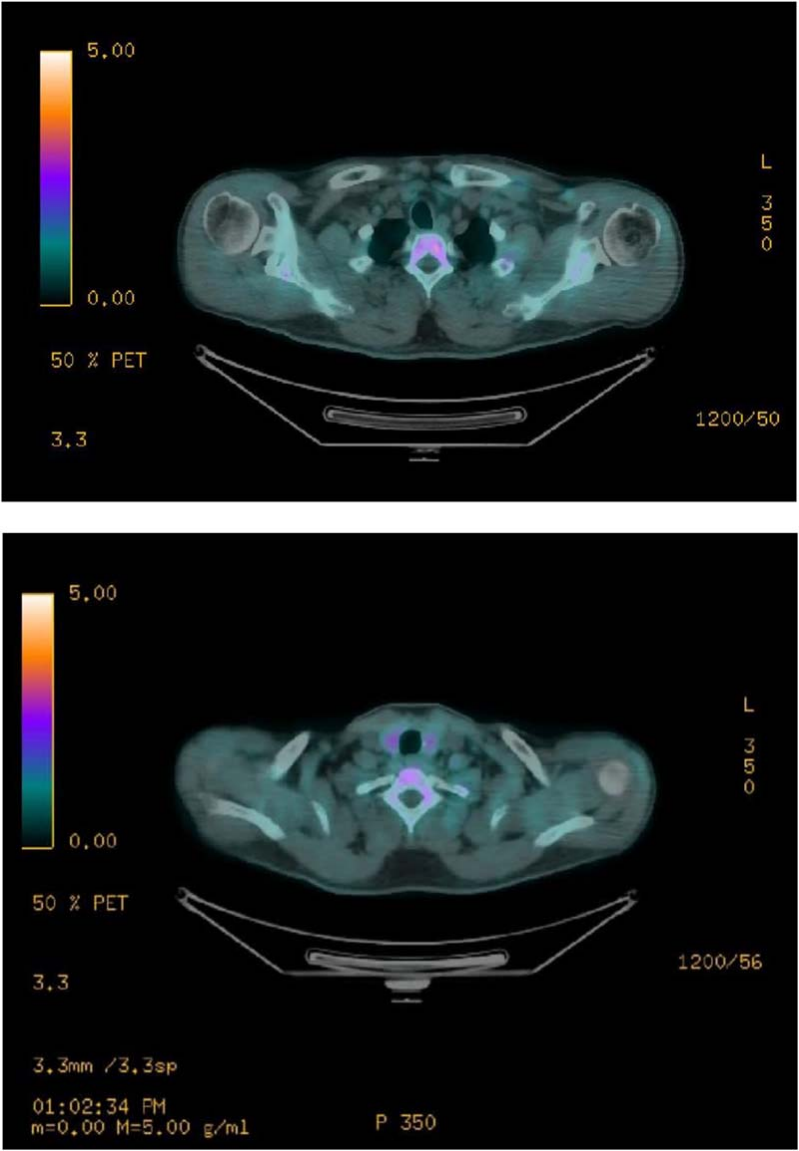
F-18 FCH PET/CT shows negative.
